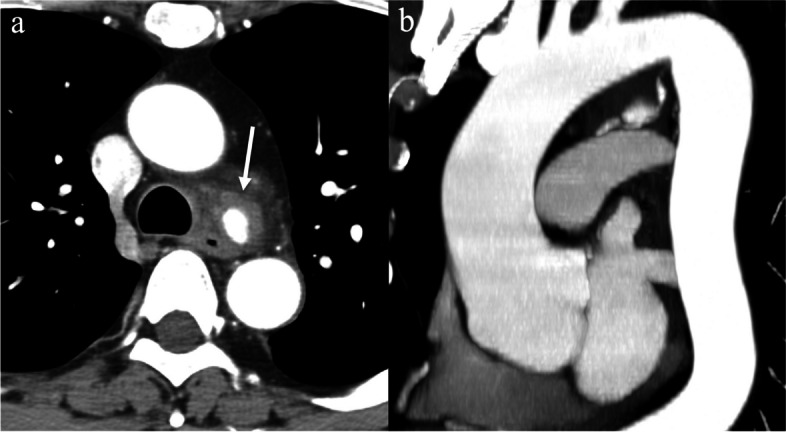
Pre-treatment imaging of the bronchial artery pseudoaneurysm. a CT angiogram axial slice demonstrating filling of the pseudoaneurysm between the descending thoracic aorta and trachea with surrounding haematoma. b CT angiogram oblique coronal maximal intensity projection (MIP) reconstruction showing the pseudoaneurysm inferior to the aortic arch
